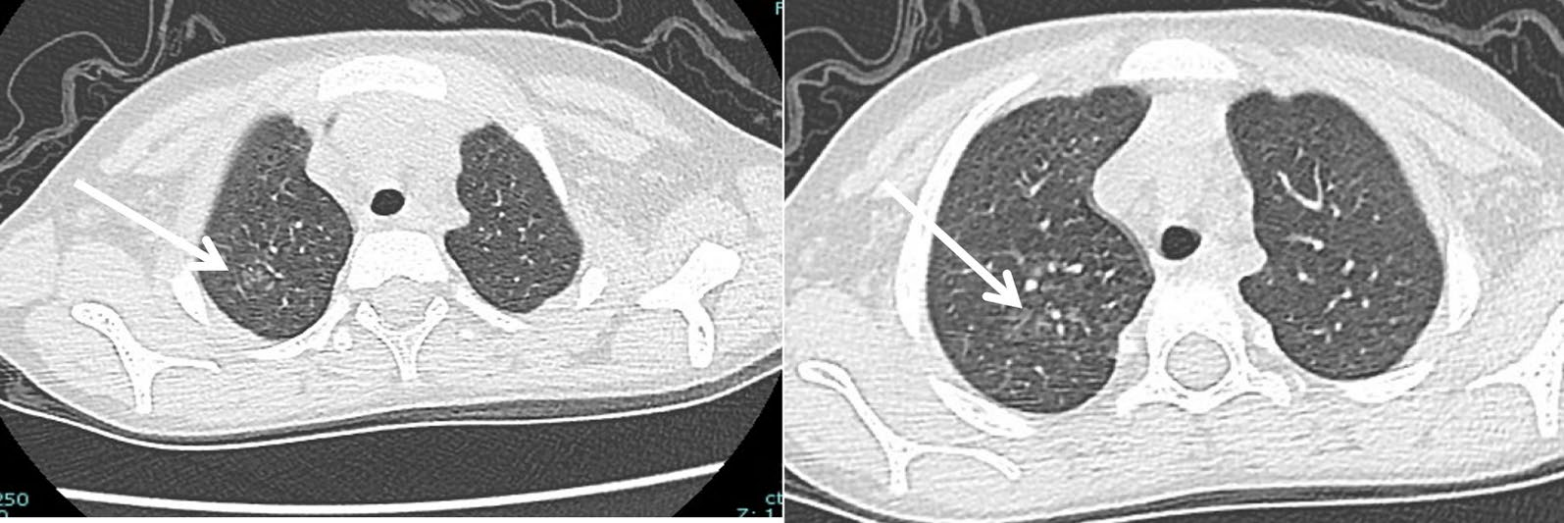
A 12-year-old male child, known case of leukemia, presented with fever for 4-day duration with mild respiratory manifestation and PCR positive; CT shows unilateral upper lobar centrilobular ground glassing opacities, CT picture is indeterminate for COVID-19, and CT severity score was 3/20
